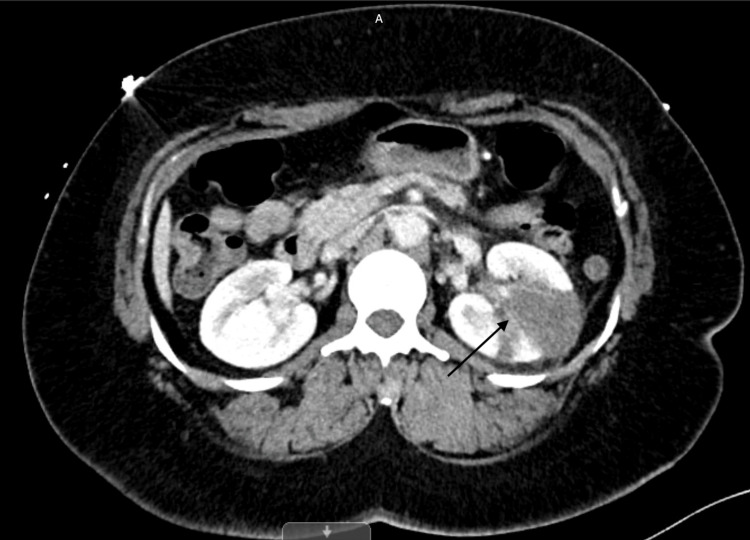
CT of the abdomen and pelvis with IV contrast Black arrow showing wedge-shaped infarction in left kidney.
